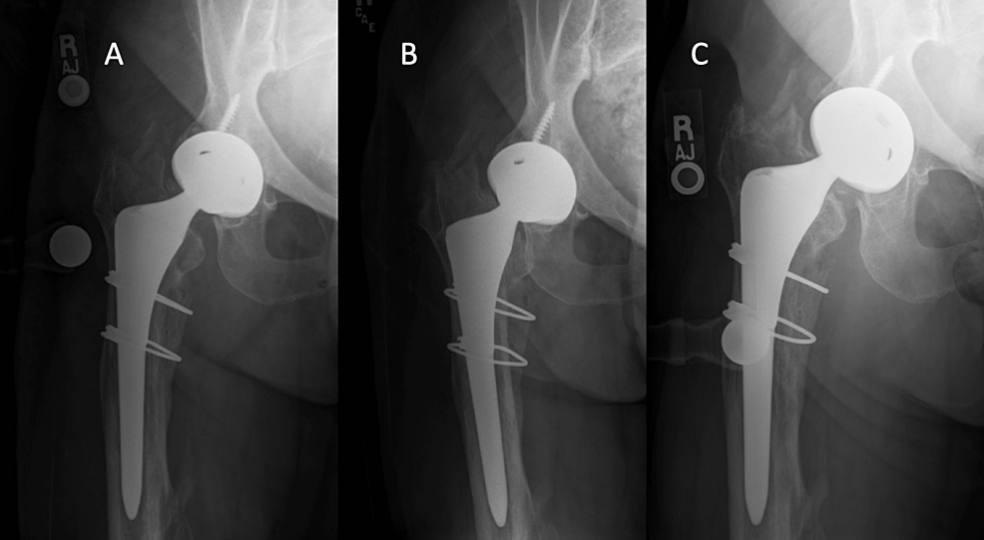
Follow-up postoperative radiographs Radiographs of the right hip taken at 4 weeks (A), 3 months (B), and 6 months (C) after surgery demonstrating stable implant subsidence without propagation of the intraoperative fracture and stability of the linear lucency.
